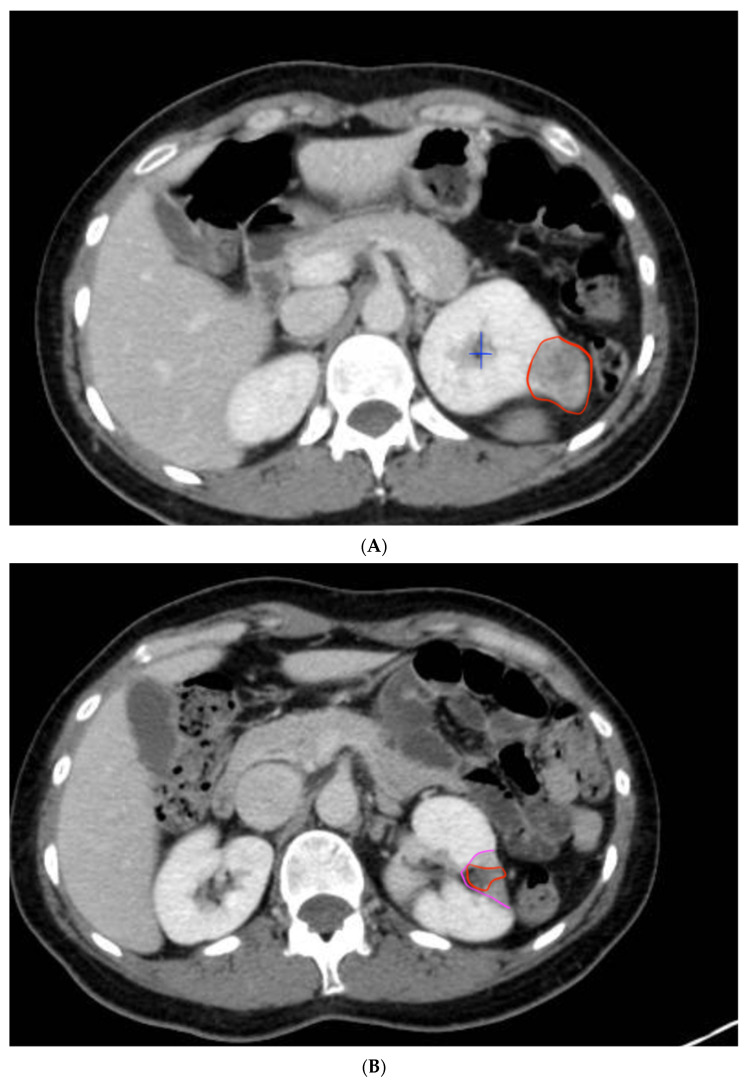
Kidney with exophytic tumor and control CT one year after surgery. (A) The first image shows a renal tumor on the posterior surface of the left kidney, measuring 3 cm, with characteristics of clear cell renal carcinoma (encircled in red). It is partially exophytic, located away from the renal hilum and the urinary collecting system. The blue cross marks the renal collecting system. (B) The second image shows the absence of the previously described mass. Post-operative changes are evident, with a scar in the area where the lesion was previously located and the morphological alterations of the kidney. Encircled in pink, the peritumoral necrotic halo is visible. In red, the bed where the tumor was located can be seen.
